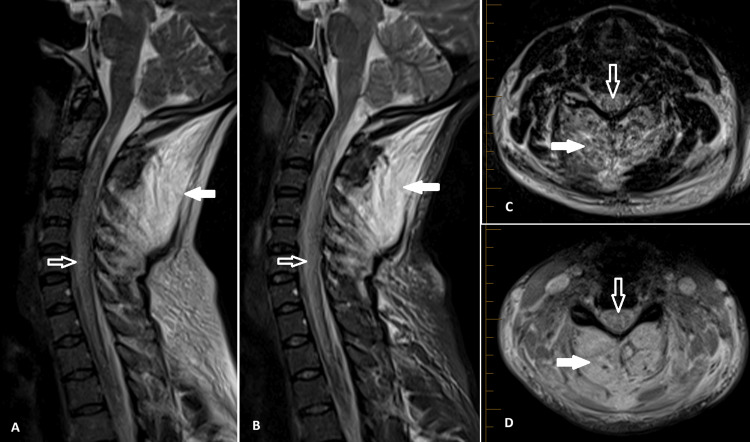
Multi-sequence cervical spine MRI demonstrating extensive paraspinal muscle edema and longitudinal cord edema (C2–T3) Multi-sequence cervical spine MRI, performed using standard clinical imaging protocols, demonstrating extensive paraspinal muscle edema and longitudinal cord edema (C2–T3). Scanner field strength (1.5T vs 3T) was not available in the medical record. A: sagittal T2; B: sagittal Short Tau Inversion Recovery (STIR); C: axial T2; D: axial Gradient Recalled Echo (GRE) images showing marked T2 and STIR signal hyperintensity in the posterior paraspinous musculature and soft tissues compatible with extensive edema (solid/white arrow), most pronounced in the multifidus muscles, suggestive of rhabdomyolysis. There is also marked T2 and STIR signal hyperintensity extending from C2 to the T3 level, indicating extensive cord edema (hollow/black arrow) with cord expansion.
